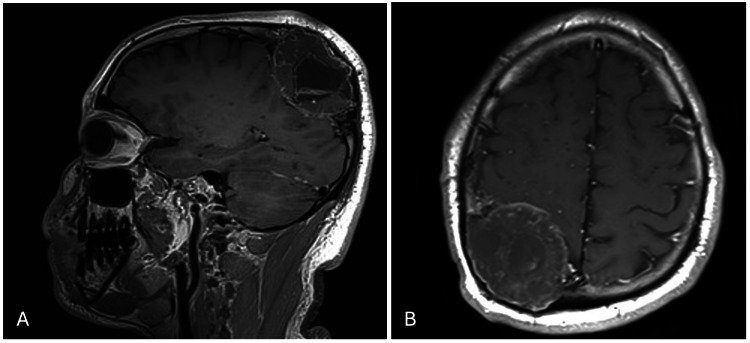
Preoperative contrasted T1 weighted image magnetic resonance imaging (MRI). Both sagittal (A) and axial (B) planes show right parietal heterogeneously enhancing lesion with an internal cystic component.
